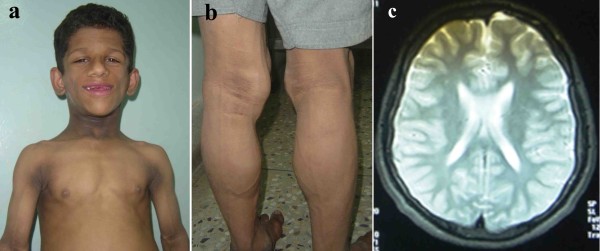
Clinical features of the patient (IV-1) with congenital generalized lipodystrophy. (a) Note curly scalp hair, hypodontia, abdominal distension, acanthosis nigricans, velvety thickening and hyperpigmentation of the skin around neck, in axillae and antecubital fossae (b) Hypertrophic muscles over calves and acanthosis nigricans in the popliteal fossae (c) Magnetic Resonance Imaging showing no exaggeration of the external or internal cerebrospinal fluid (CSF) spaces suggesting that brain atrophy is not evident.
